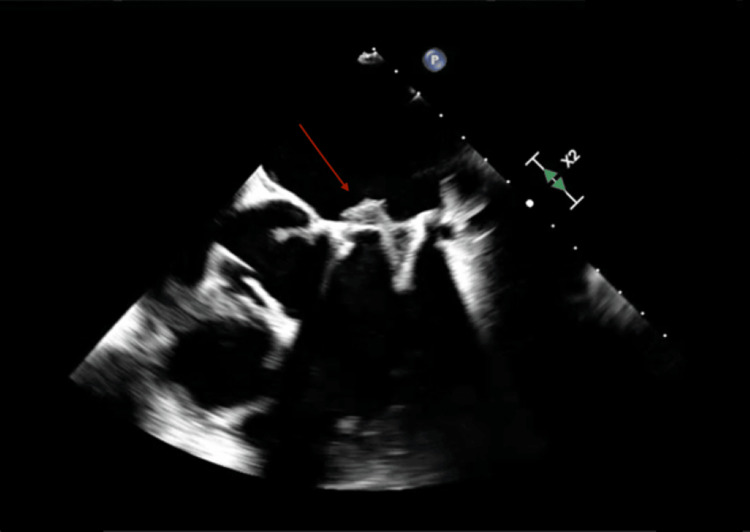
Transesophageal echocardiography with evidence of large vegetation on the anterior right cusp indicated by the red arrow.
